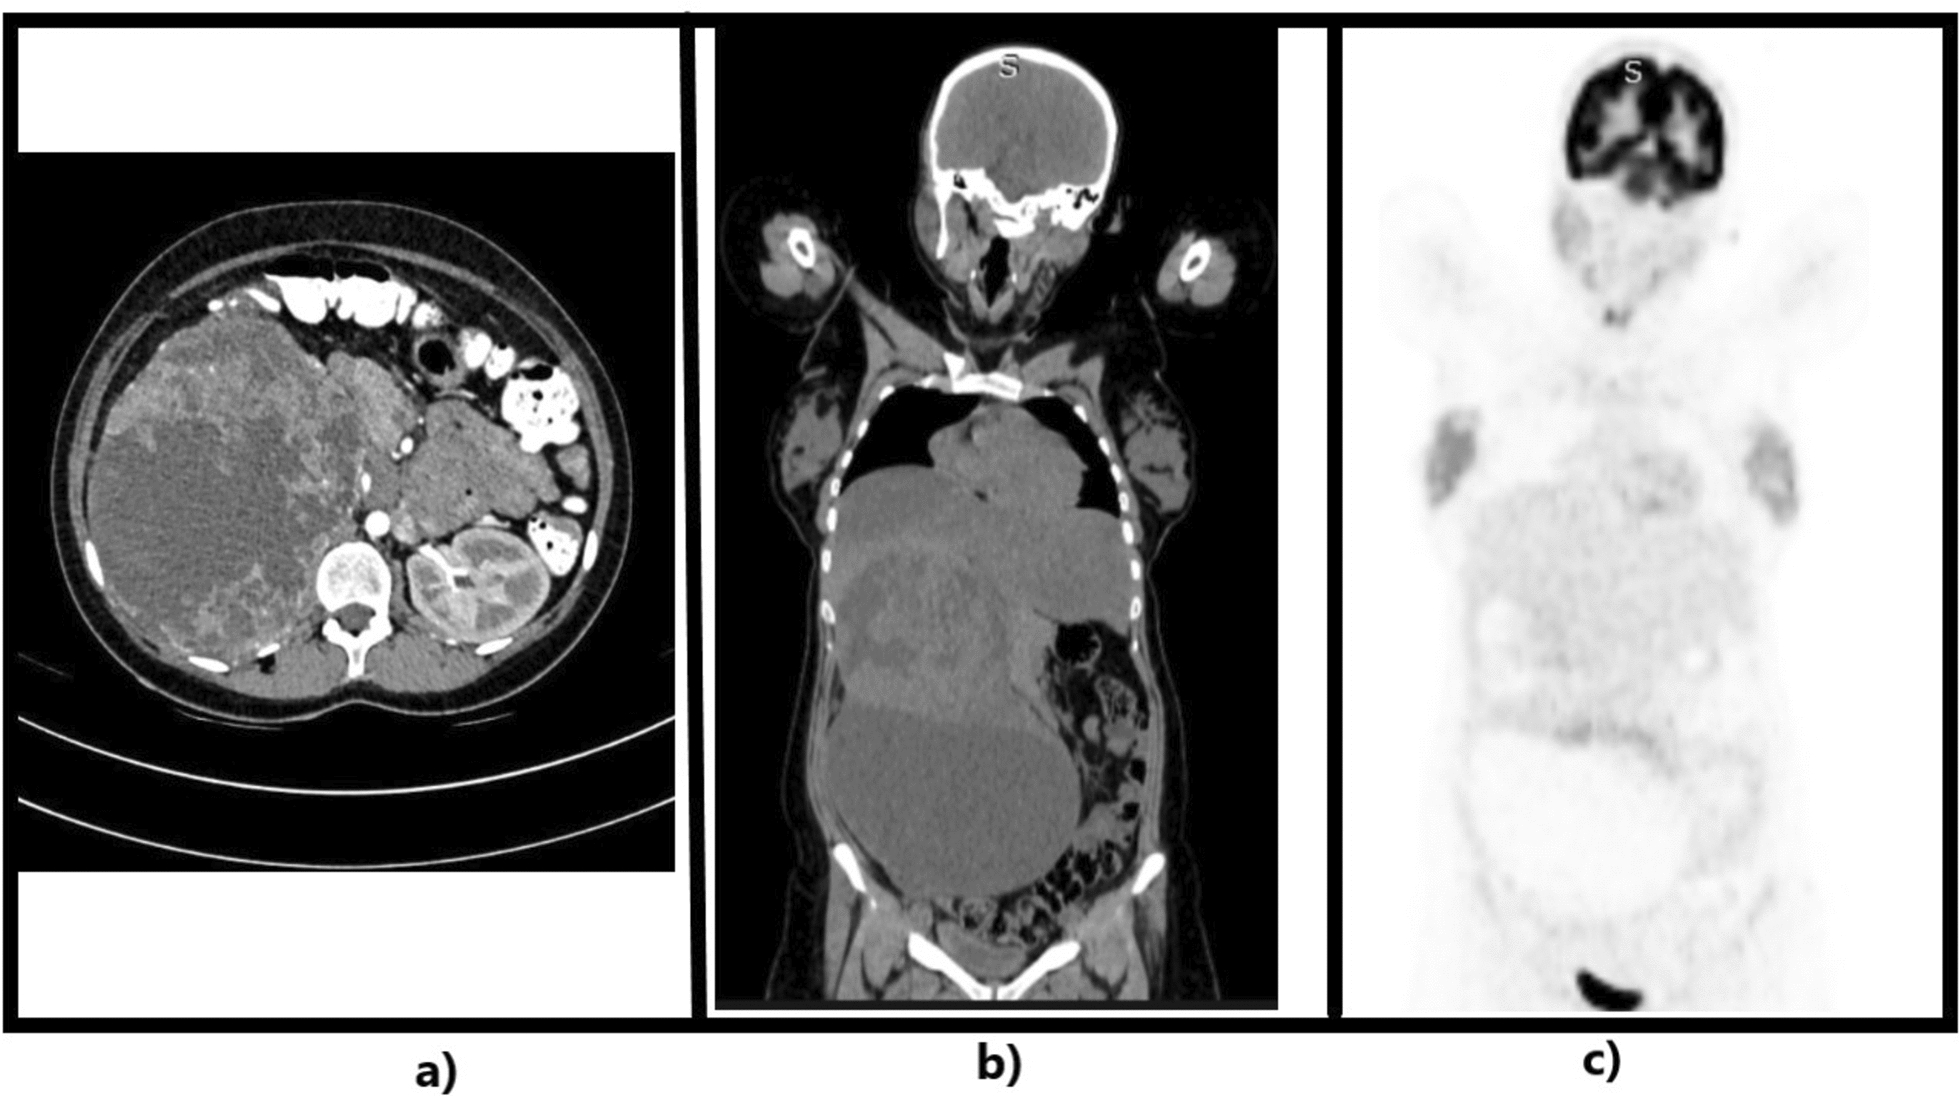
a Axial contrast enhanced computed tomography films showing a mass arising from the right kidney and a normal left kidney. b Coronal contrast enhanced computed tomography films showing the extent of the tumor. c Positron emission tomography and computed tomography films showing no evidence of metastasis
